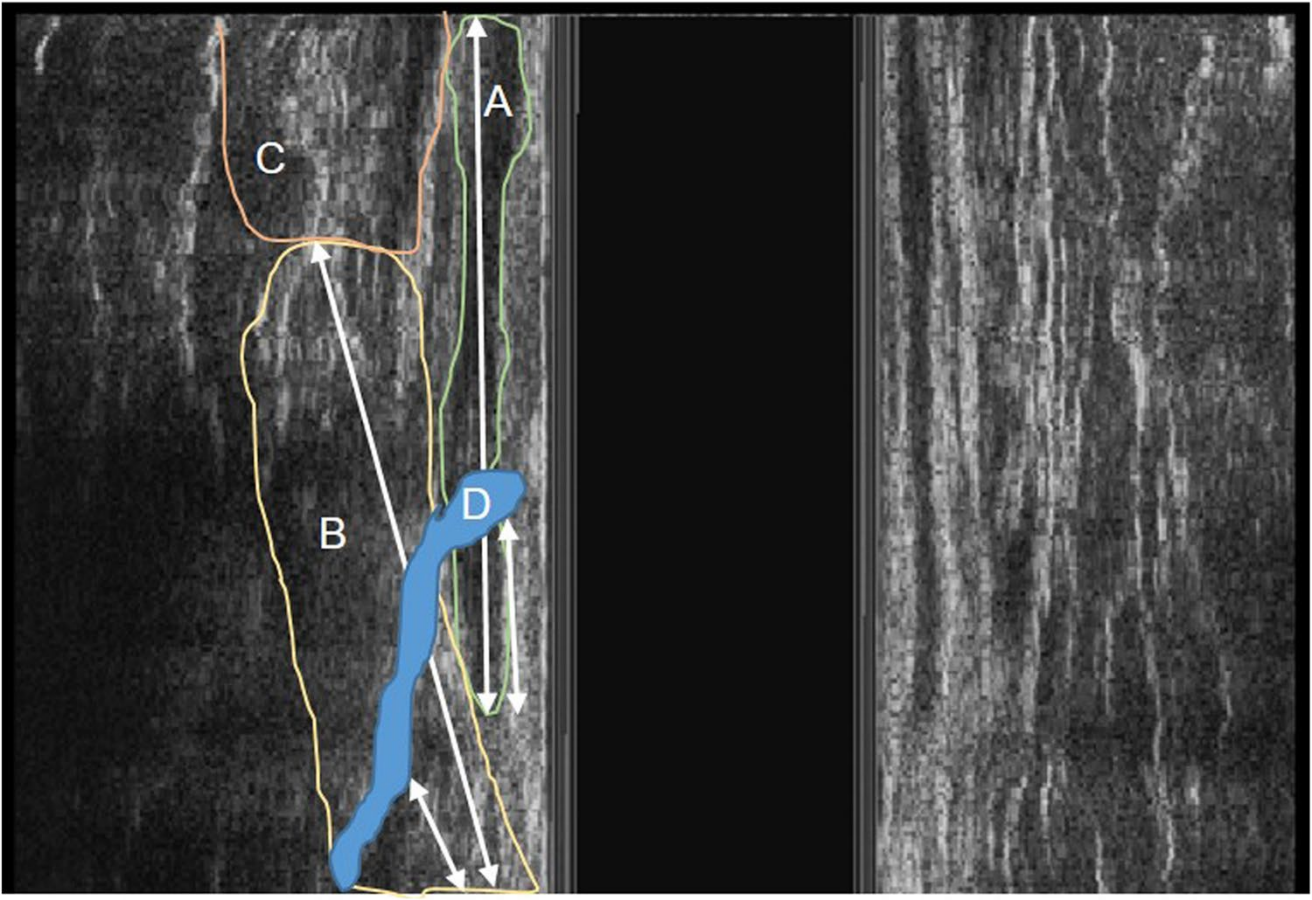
Endoanal ultrasound image showing a coronal section of a right lateral transphincteric fistula and arrows indicating total length and involved sphincter of both the internal and external anal sphincters. A internal anal sphincter, B external anal sphincter, C puborectalis muscle, D fistula tract
